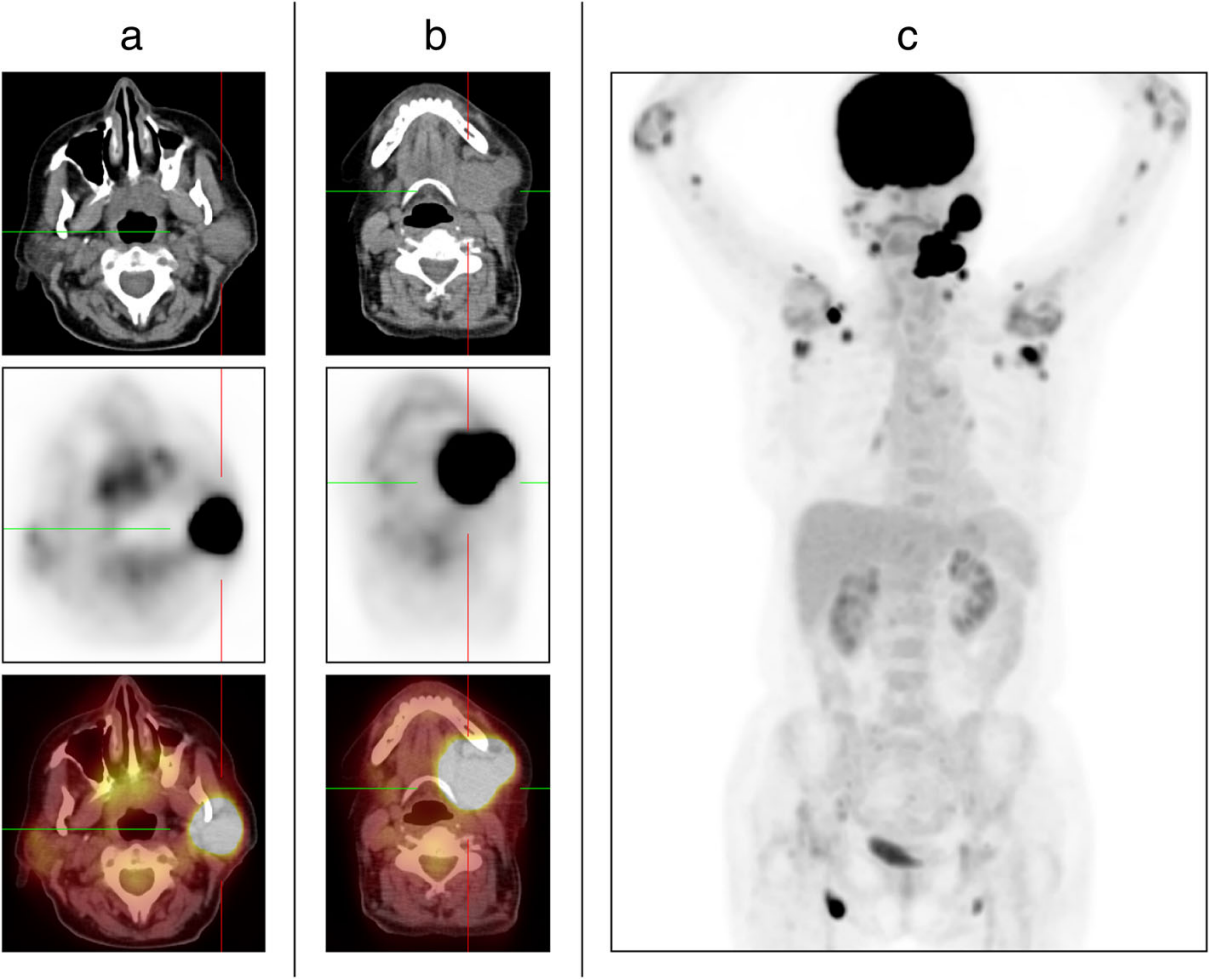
Fluorine-18 fluorodeoxyglucose (FDG) positron emission tomography combined with computed tomography. a Increased FDG accumulation in the left parotid gland. b Increased FDG accumulation in the left submandibular gland. c Multiple pathological foci of FDG uptake with maximum standardized uptake value up to 50.1
